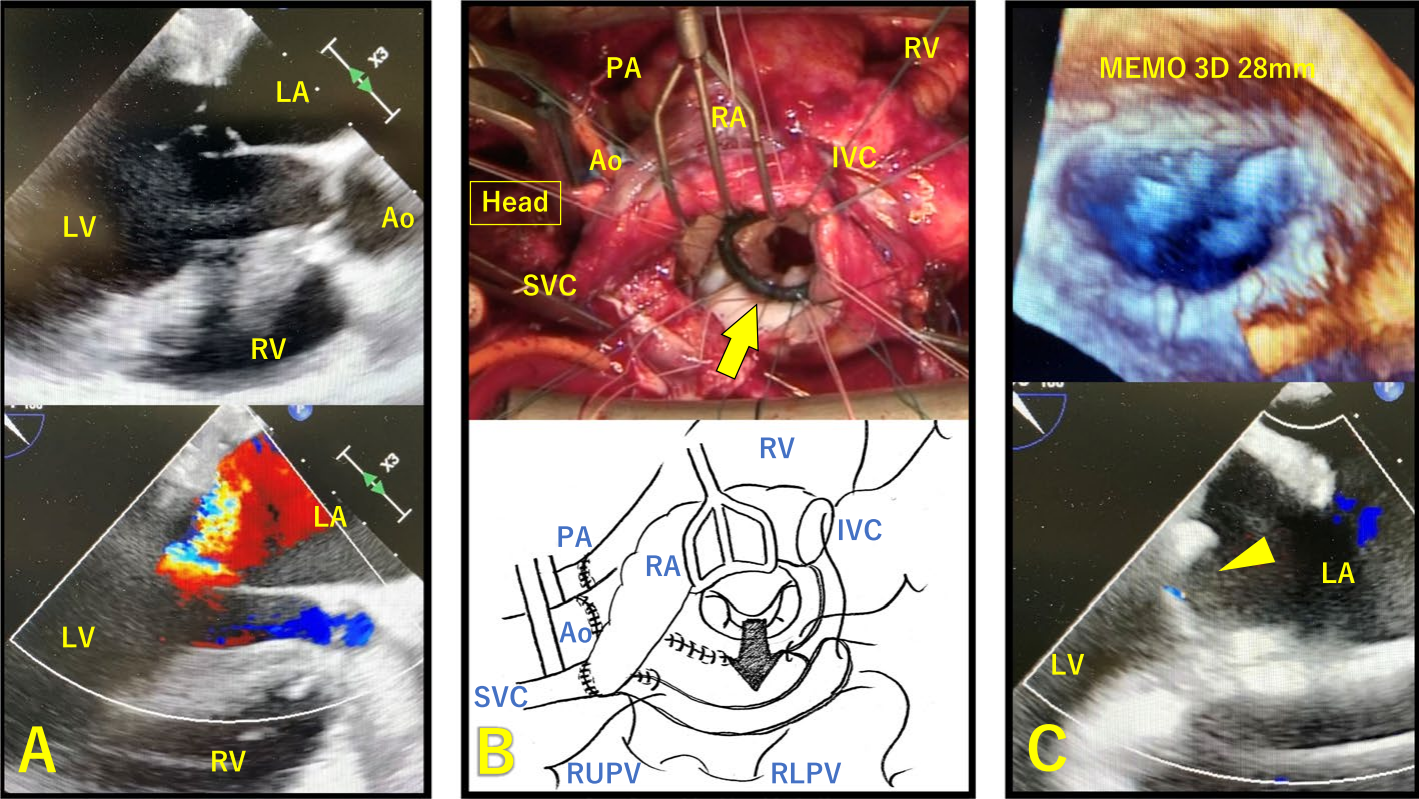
A Intraoperative TEE revealed no prolapse but showed severe MR originating from the center with mild tethering at the anterior and posterior leaflets, resulting in a shallow coaptation length. B We detached the IVC anastomosis and partially dissected the right side of the left atrial anastomosis to approach the mitral valve (arrow), obtaining satisfactory exposure. C Mitral annuloplasty was performed using one downsized 28-mm Memo 3D ring (Sorin Biomedica Cardio S.r.L., Saluggia, Italy), and TEE confirmed that the MR was well controlled (arrowhead). *TEE, transesophageal echocardiography; MR, mitral regurgitation; Ao, aorta; PA, pulmonary artery; LA, left atrium; LV, left ventricle; RA, right atrium; RV, right ventricle; SVC, superior vena cava; IVC, inferior vena cava; RUPV, right upper pulmonary vein; RLPV, right lower pulmonary vein*
